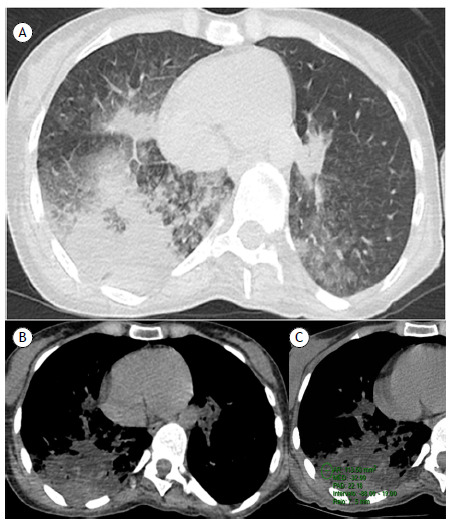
CT scan of the chest at the level of the lower lobe (with lung window settings in A and mediastinal window settings in B and C), showing heterogeneous airspace consolidation with superimposed areas of low attenuation (ranging from −88 to −19 Hounsfield units) in the posterior region of the right lower lobe. This finding is diagnostic of exogenous lipoid pneumonia.
